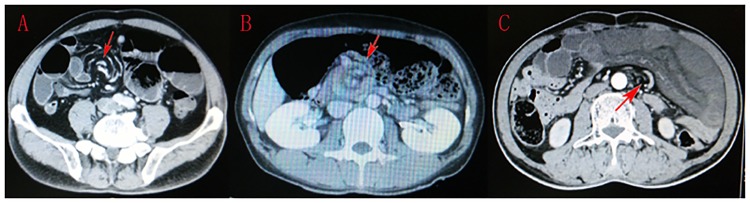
Contrast-enhanced abdominal CT demonstrated the dilation of small bowels and whirl-like pattern of distended small bowels encircling the mesenteric artery or its branches (red arrows).
